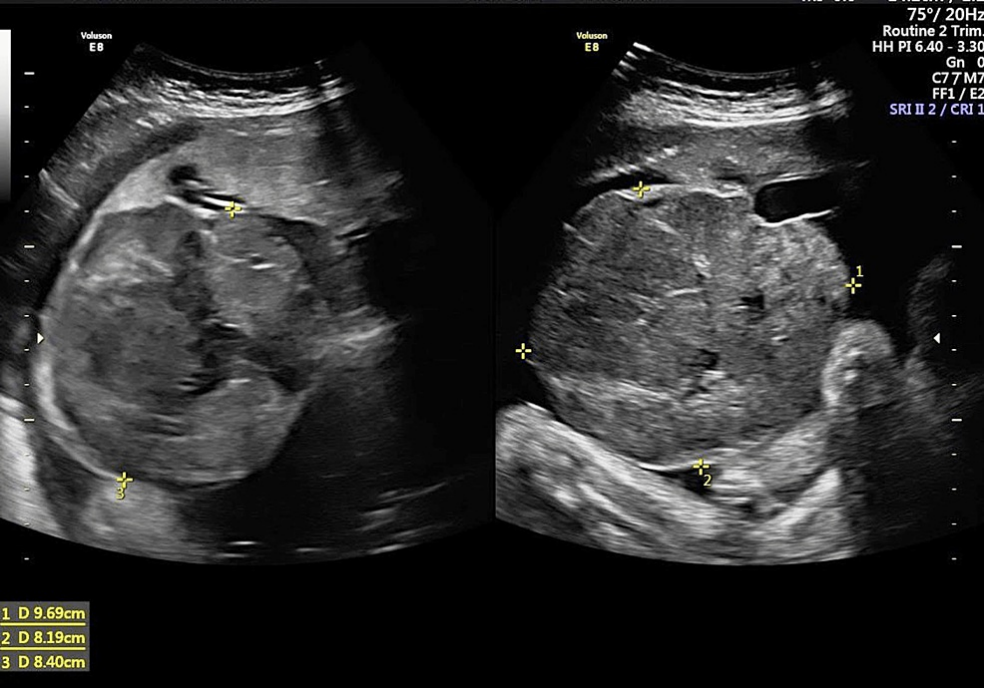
Ultrasound image at 33 weeks of gestation.
